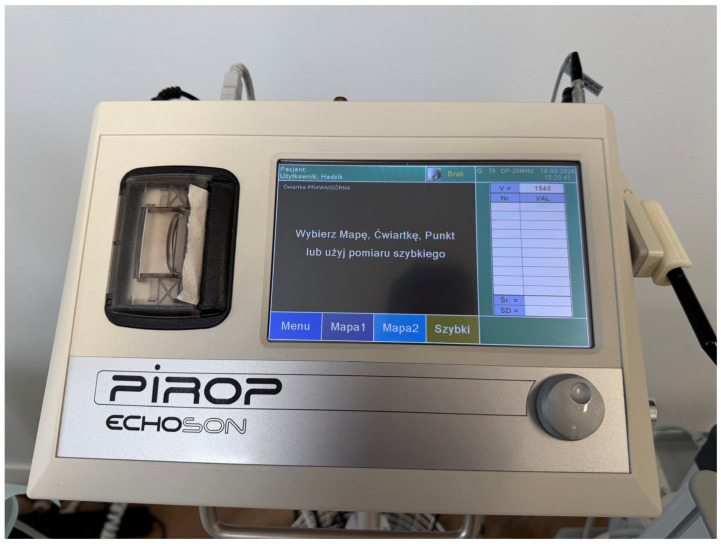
Pirop ultrasound-based biometric scanner (ECHOSON^®^, Poland) used for non-invasive quantitative assessment of peri-implant soft tissue thickness. Ultrasonic measurements were performed according to a standardized protocol and provided soft tissue thickness values expressed in millimeters.
